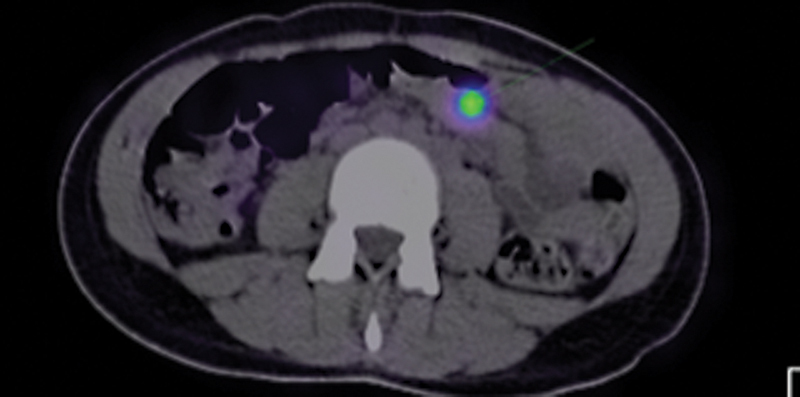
Erythrocyte-labeled scintigraphy—single-photon emission computed tomography/computed tomography (SPECT/CT) images of a patient whose Meckel's diverticulum scintigraphy pointed out gastrointestinal bleeding rather than ectopic gastric mucosa (EGM) and finally the bleeding site was determined only by SPECT/CT at splenic flexure adjacent to spleen activity.
